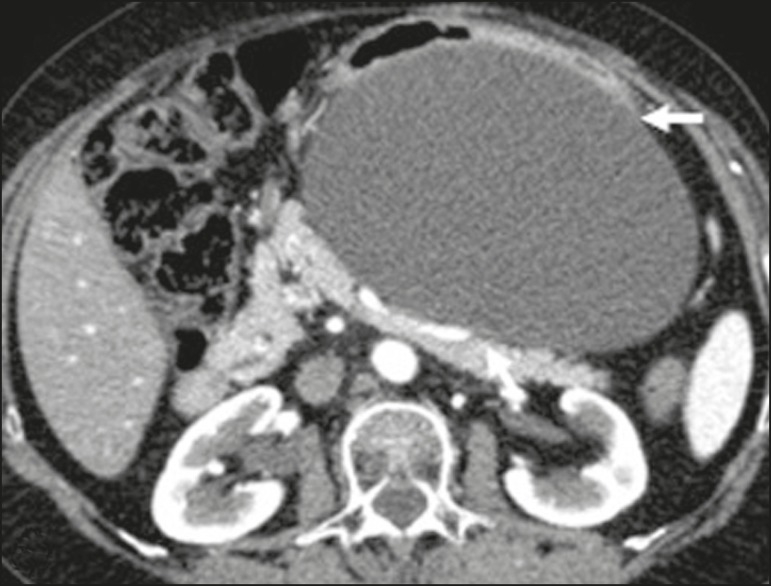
Retroperitoneal cystic lymphangioma in a 37-year-old male. CT scan
